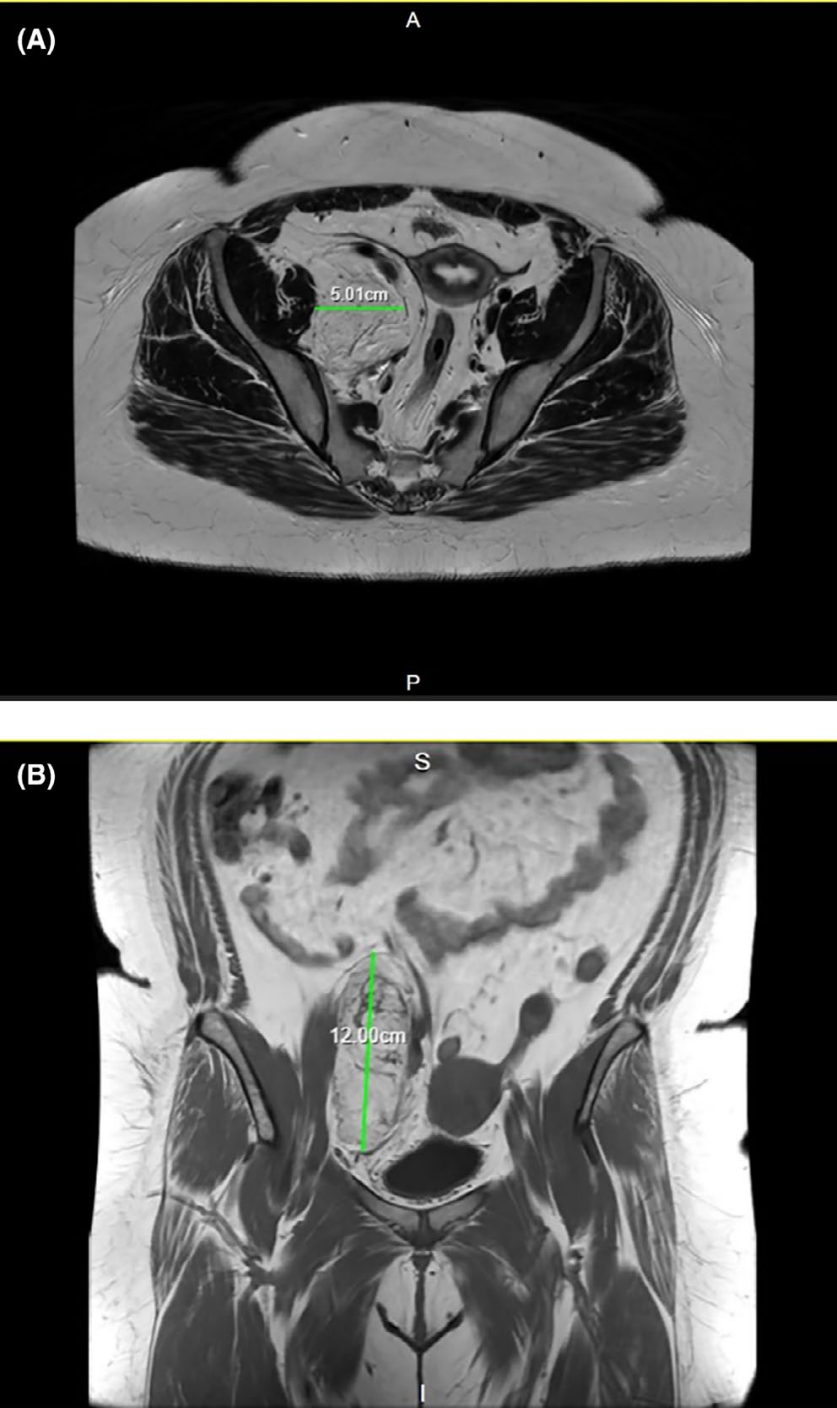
T1 weighted MRI scan demonstrating a large lipomatous mass measuring 120 × 50 × 75 mm medial to the right iliopsoas, displacing the iliac vessels, uterus and bladder without invasion of adjacent muscles and organs. (A) Axial view. (B) Coronal view.
